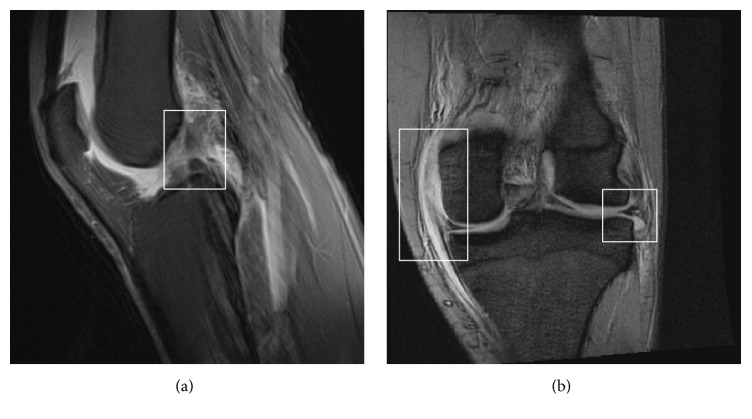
(a) MRI showing a torn anterior cruciate ligament. (b) MRI showing medial collateral ligament injury and displacement of the lateral meniscus.
